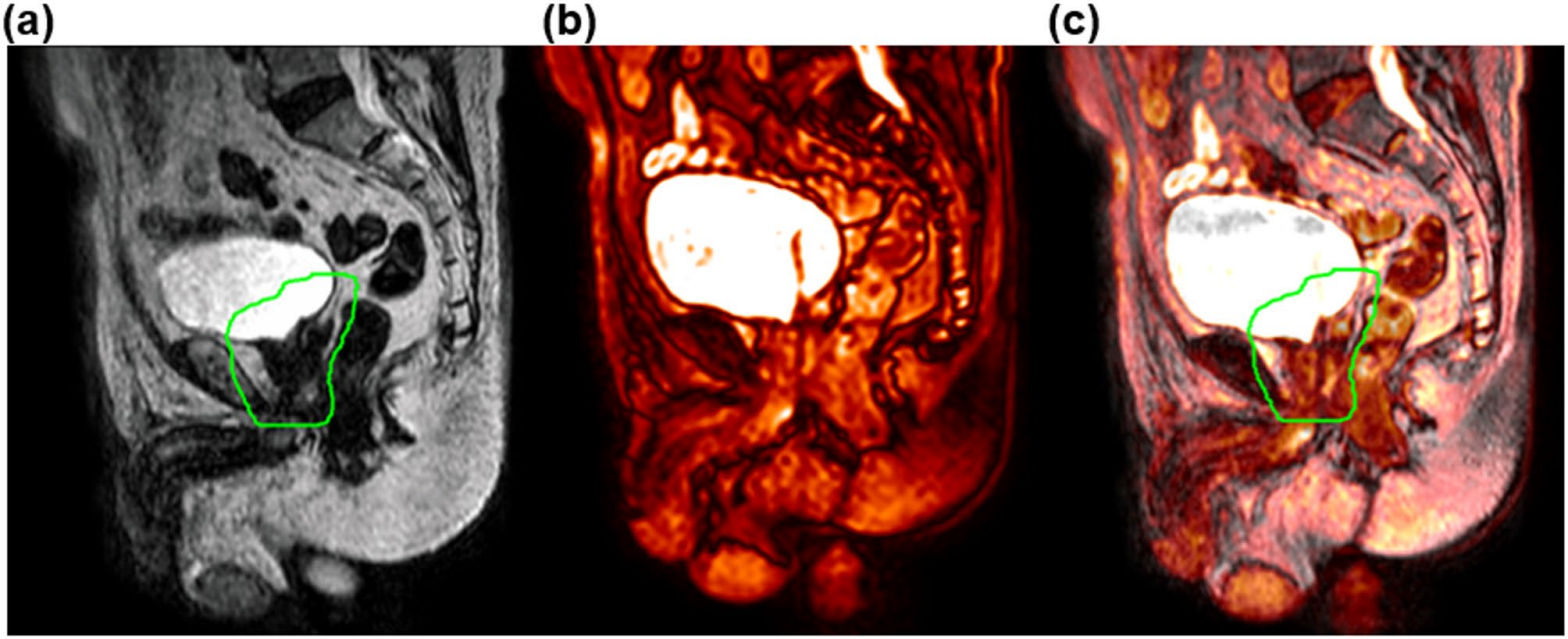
(a) Daily MR images included PTV structure (green line), (b) the converted 2D cine MR images, and (c) registration of two images in the sagittal planes.
